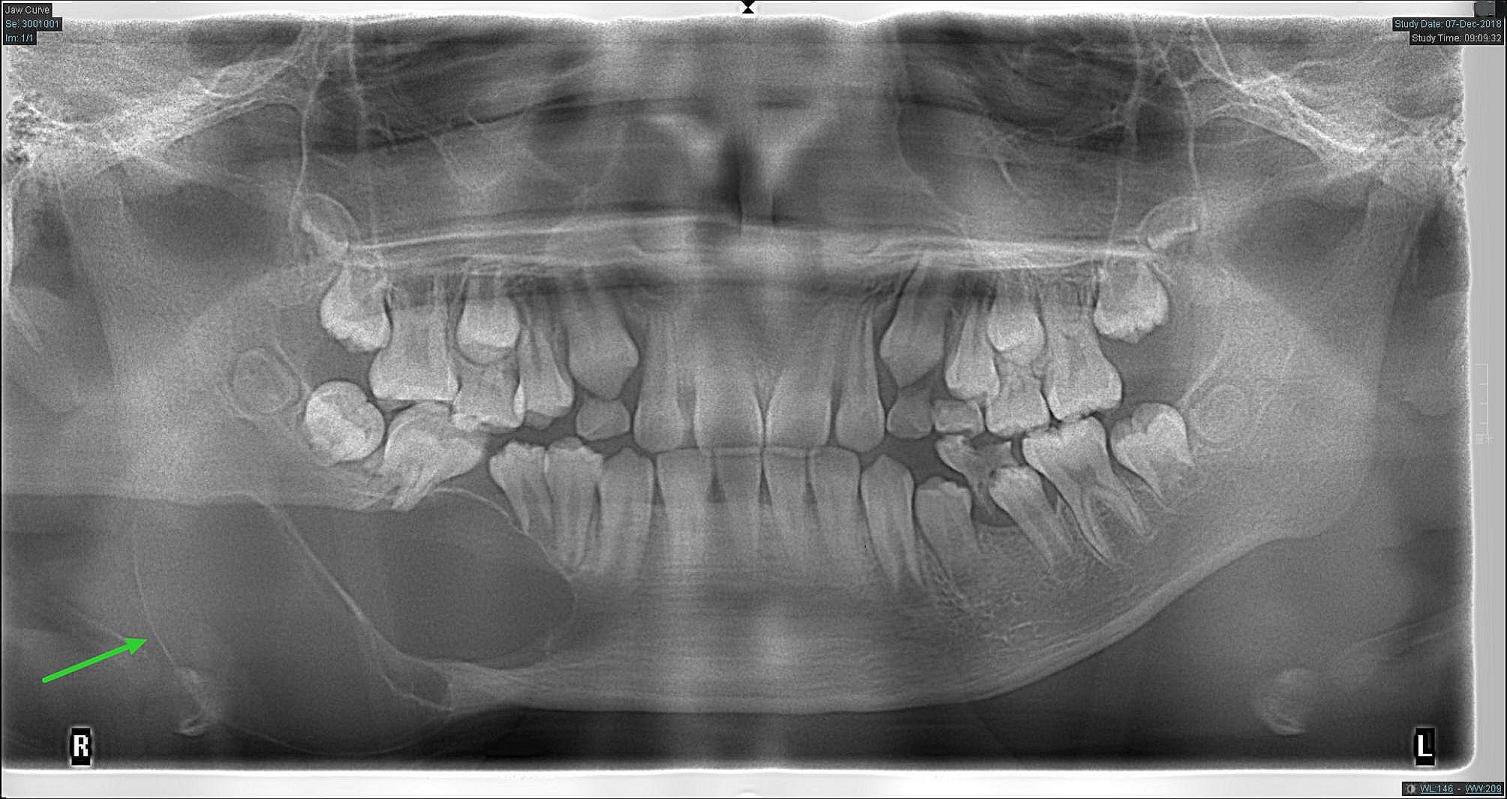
Preoperative panoramic radiography showing a typical AM in the right mandible
